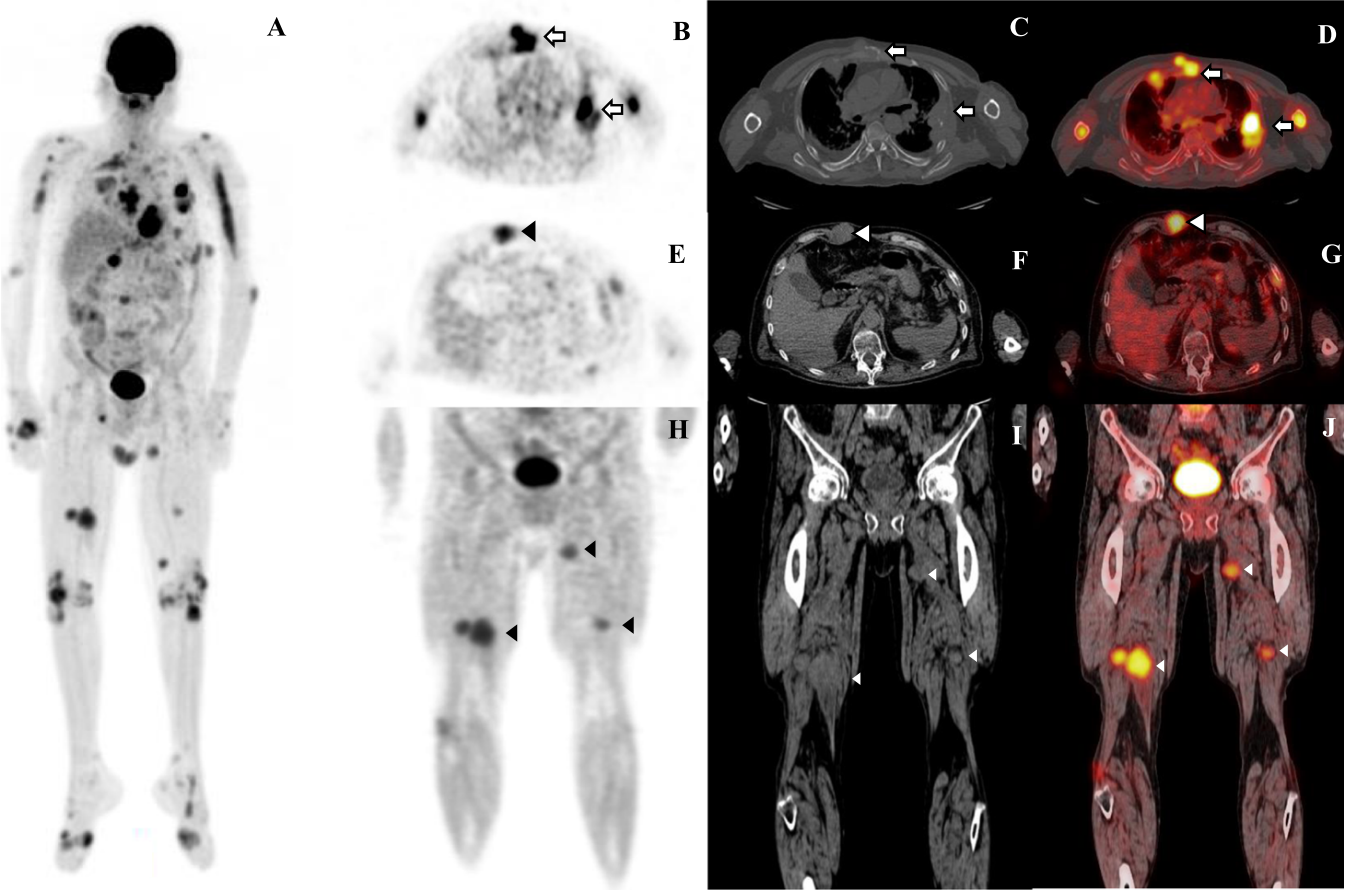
a MIP image shows multiple soft tissue masses with high FDG uptake and hypermetabolic lytic bone lesions. b-d On axial images, lytic lesions in sternum and ribs with soft-tissue mass and increased FDG uptake (white arrows). e-g Hypermetabolic subcutaneous mass in right abdomen wall with FDG uptake (arrowheads). h-j In extremities, subcutaneous lesions with high uptake of FDG were seen (arrowheads)
